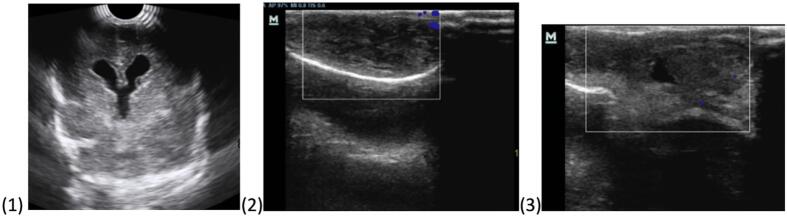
Cranial ultrasound revealed dilated lateral ventricles with a soft tissue mass with some fluid content at the occipital lobe in continuation with the underlying brain through a defect in the skull bone.
